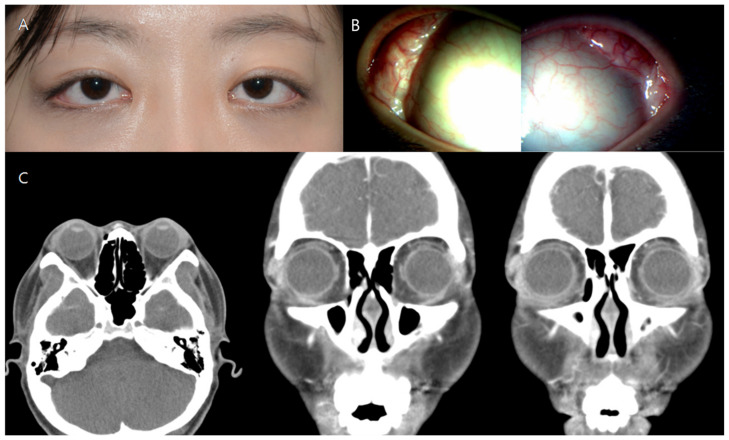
Preoperative images of Case 1. Both upper lids exhibited swelling (A) along with a protruding palpebral lacrimal lobe (B). Computed tomography showed a heterogeneous contrast-enhanced inflammatory lacrimal gland mass in both lacrimal glands (C).
